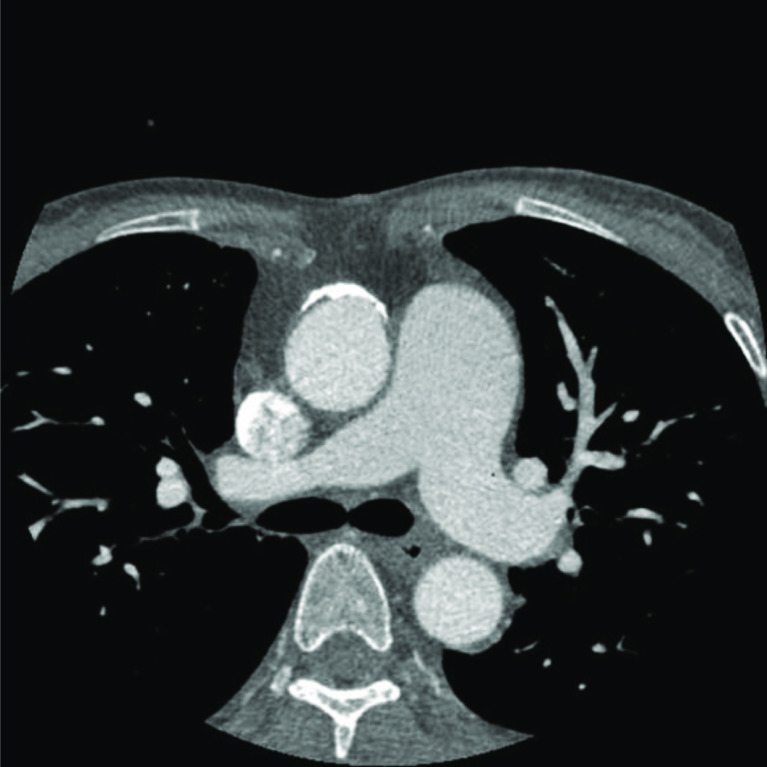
Preoperative contrast-CT indicates patch repair for pseudoaneurysm of the ascending aorta. CT: Computed Tomography.
